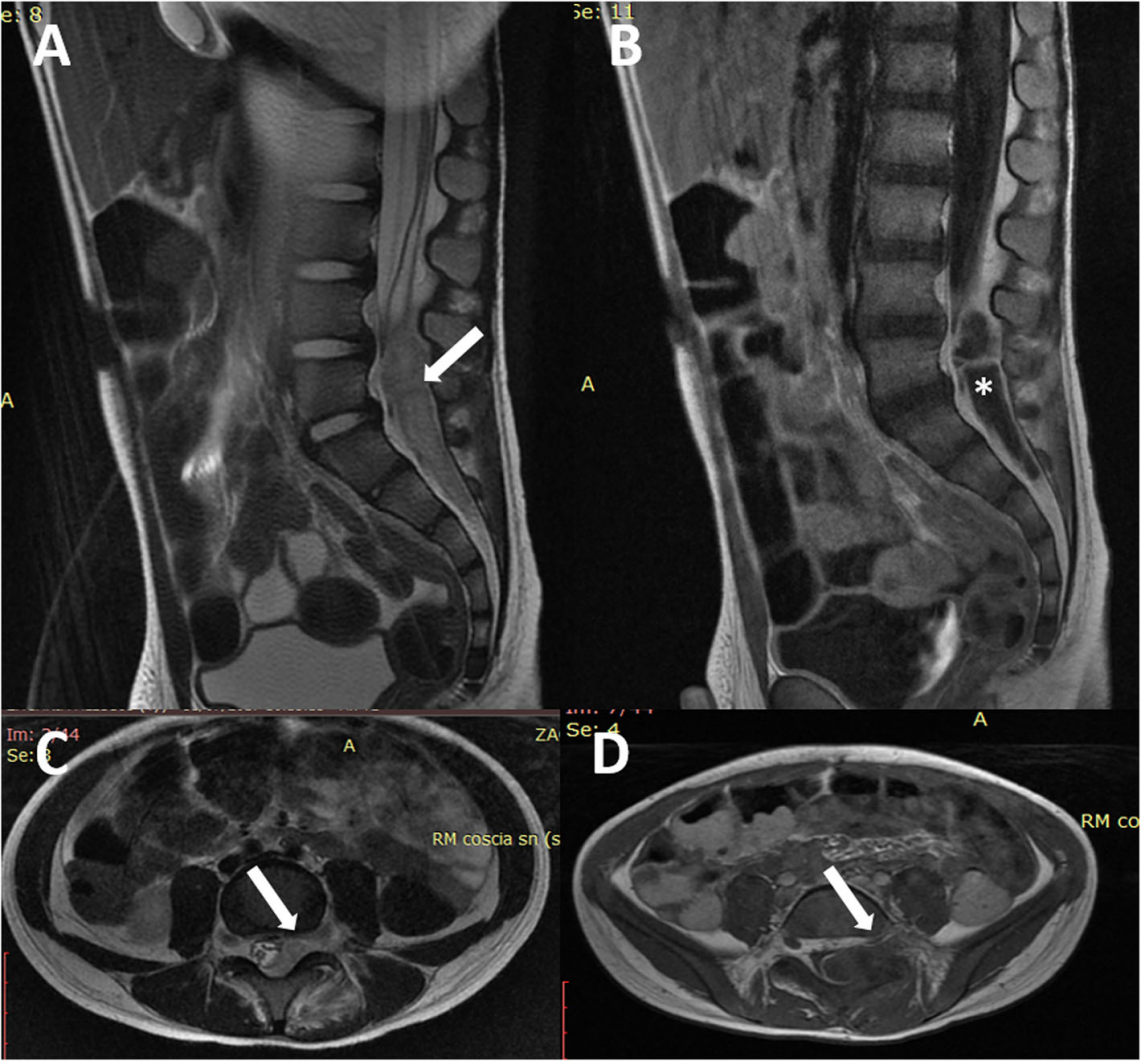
Preoperative MRI scan of case #1. An epidural abscess (L4-S2) is evident on sagittal T2w (a, arrow) and gadolinium T1w (b, asterisk). The respective axial images (c, d) show the compression on the dural sac and the involvement of the left L4-L5 foramen (arrow)
